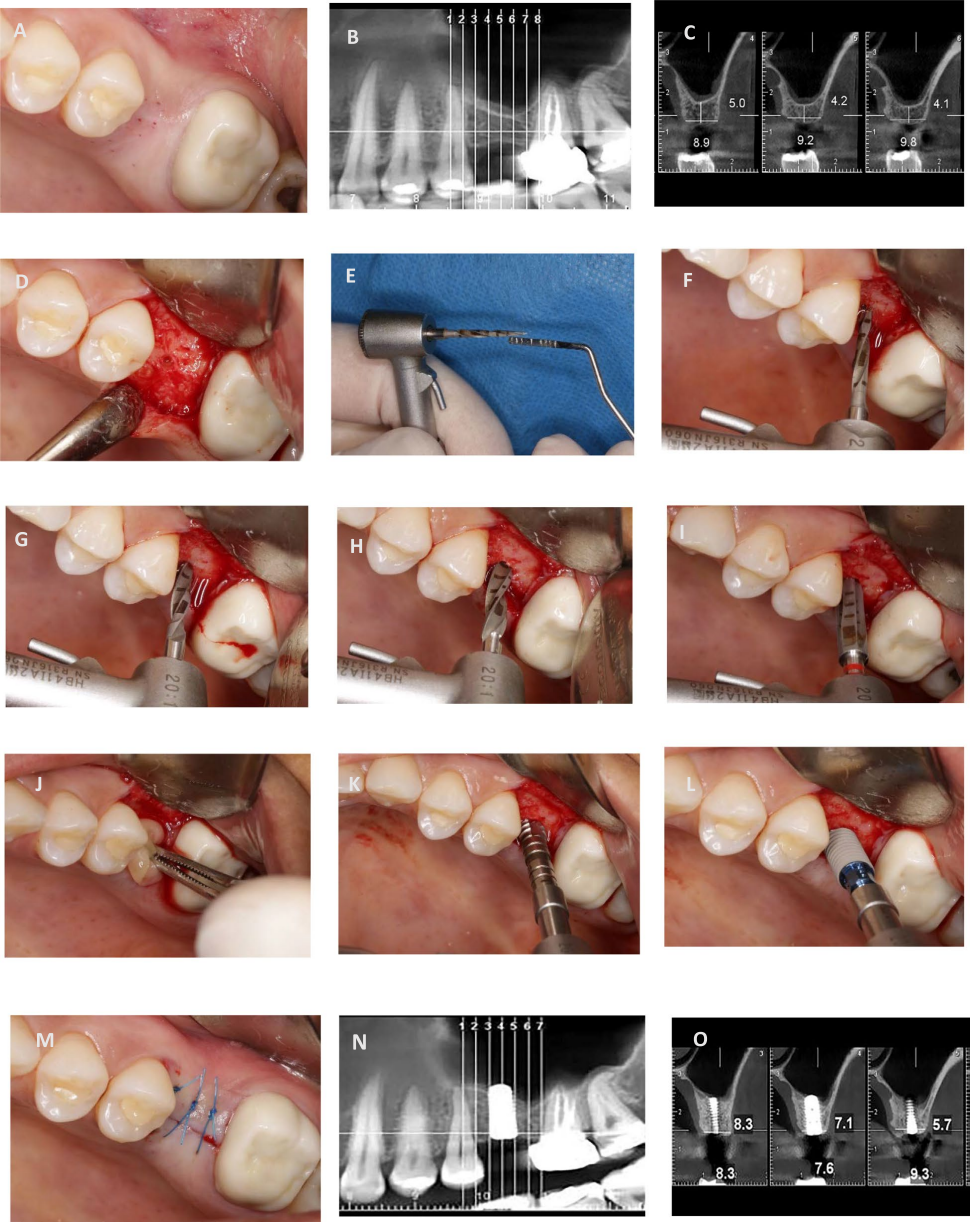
Case 2. (A) Pre‐operation view. (B, C) The ideal position of the implant. (D) Mid‐crestal incision. (E, F) Penetration rate of starter drill within the close distance of the cortical bone of the sinus floor. (G) Inserting a drill with a diameter of 2.2 mm, in the same manner as the starter drill. (H) Inserting a 2.8‐mm‐diameter drill based on RBH to perforate the cortical bone of the sinus floor. (I) Inserting a 3.5‐mm‐diameter taper‐line drill based on RBH to expand the sinus floor hole. (J) Inserting PRF into the osteotomy hole. (K) The sinus membrane was elevated by transferring PRF using a bone tap drill into the sinus cavity. (L) Implant insertion (4.1 × 8 mm, BL). (M) Suturing (Nylon 5‐0). (N, O) CBCT after 5 months.
